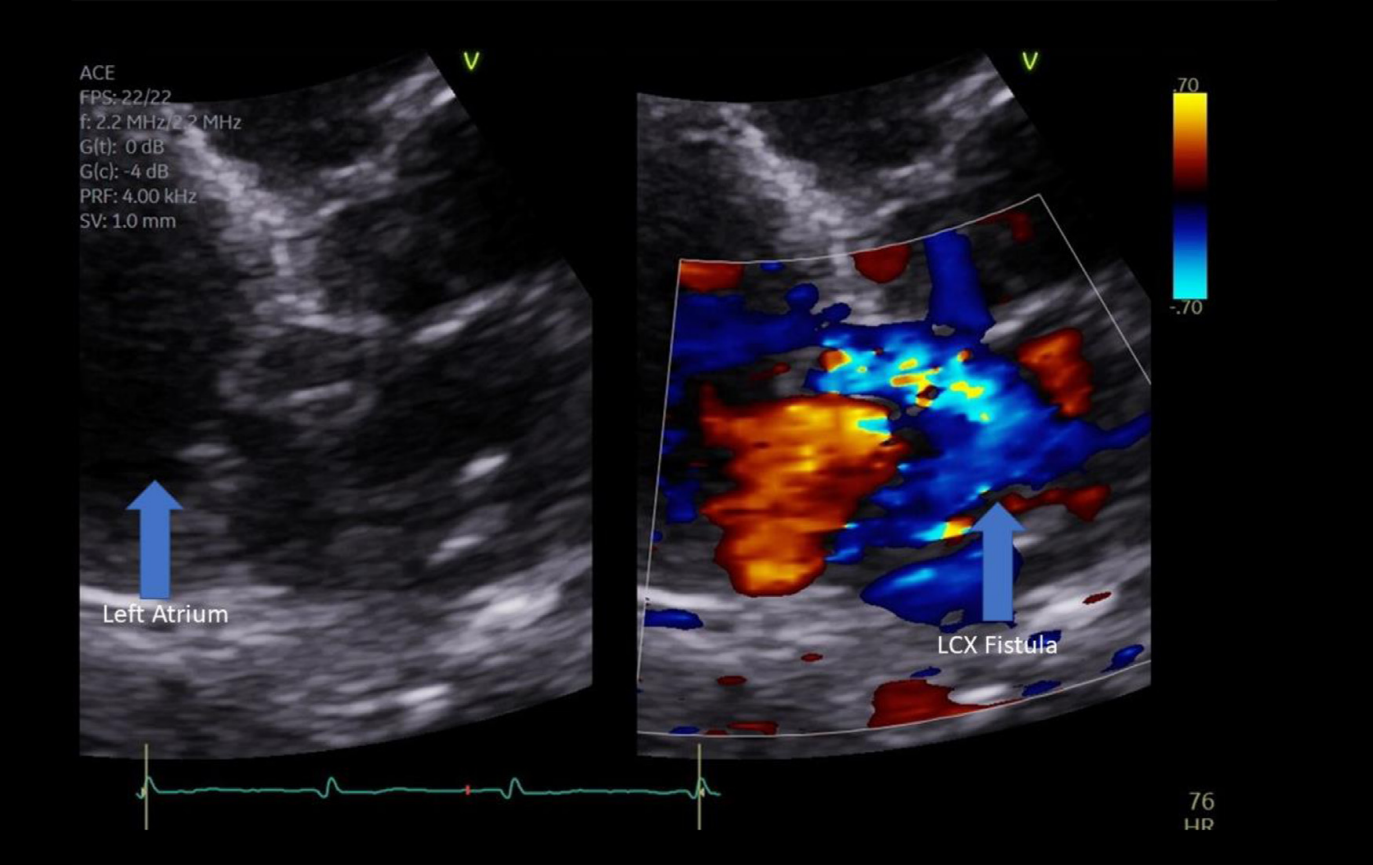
Grayscale and Doppler echocardiogram: turbulent flow from the LCX CAF (blue arrow in the right image) connecting to the left atrium (blue arrow in the left image).
